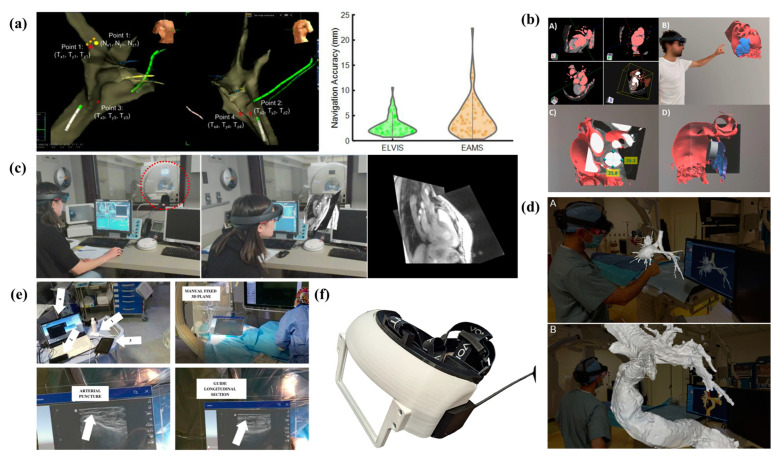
(a) (Left) target Point 1 (Tx1, Ty1, Tz1) and the marked catheter location Point 1 (Nx1, Ny1, Nz1) during a first-in-human clinical study. (Right) Navigation accuracy using ELVIS and EAMS [4]. (b) Workflow for holographic model generation and analysis involves (A) Creating an endocardial isosurface for the 3D model, (B) Visualizing the model using the MR application, (C) Conducting quantitative morphology analysis to identify the maximum and minimum diameters of the landing zone; (D) virtually implanting the LAA occluder into the holographic model [5]. (c) Operation of the complete system at the MR scanner: (Left) A user wearing a mixed-reality headset sits at the scanner control computer, preparing to scan a healthy volunteer inside the scanner bore (outlined with a red dashed circle). (Middle) During the scan, the user observes a real-time multi-slice rendering of the volunteer’s heart. (Right) The user’s view of this rendering [1]. (d) Side-by-side comparison of augmented reality and computer models of reconstructed 3DRAs post Hemi-Fontan operation, including airway (A) and a magnified intraluminal view post-complex Fontan palliation (B) [7]. (e) Different views of the system: The top-left quadrant displays the main hardware components of the transmitter module: (1) ultrasound scanner, (2) video capture card, (3) tablet, and (4) laptop. The top-right quadrant shows a plane rendering of ultrasound images, manually aligned by the doctor using a button located in the top right corner of the window (next to the close button). The bottom-left quadrant depicts an arterial puncture assisted by the system, while the bottom-right quadrant shows the guide inserted into the artery, viewed from a longitudinal section [6]. (f) Mixed reality head-mounted display used in the study [3].
